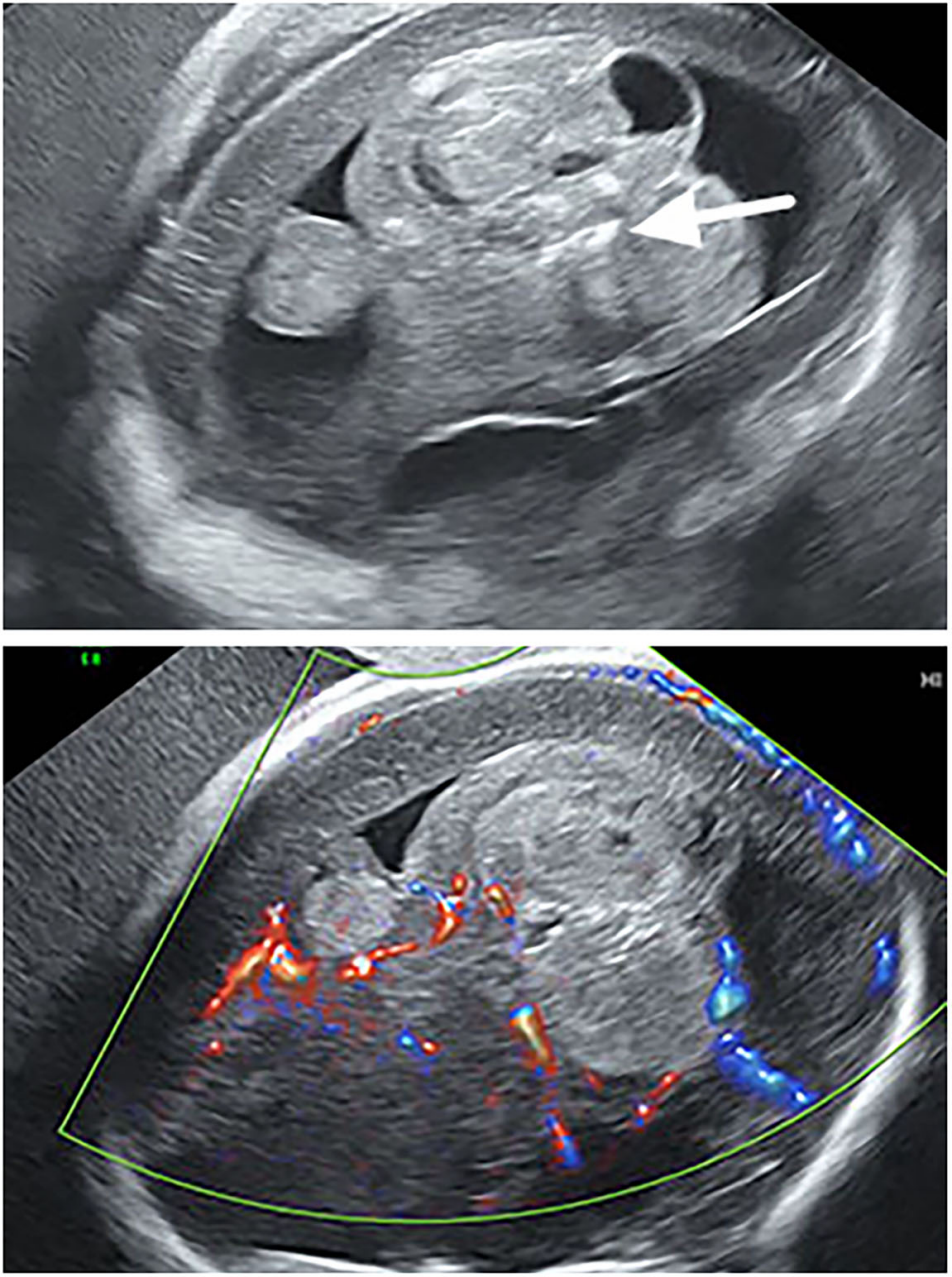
Prenatal ultrasound findings. (A) The intracranial mass contains multiple hyperechoic foci (long arrow). (B) CDFI demonstrates relatively abundant intralesional vascularity.
